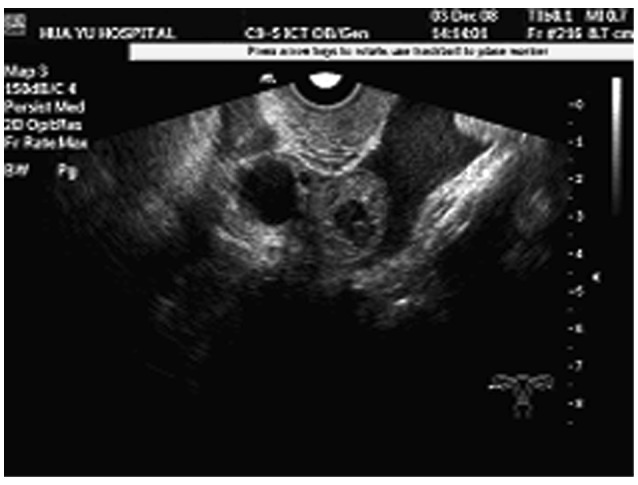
Ultrasound showing a 6.9×4.6-cm mixed echo mass in the left side of the pelvic cavity.
